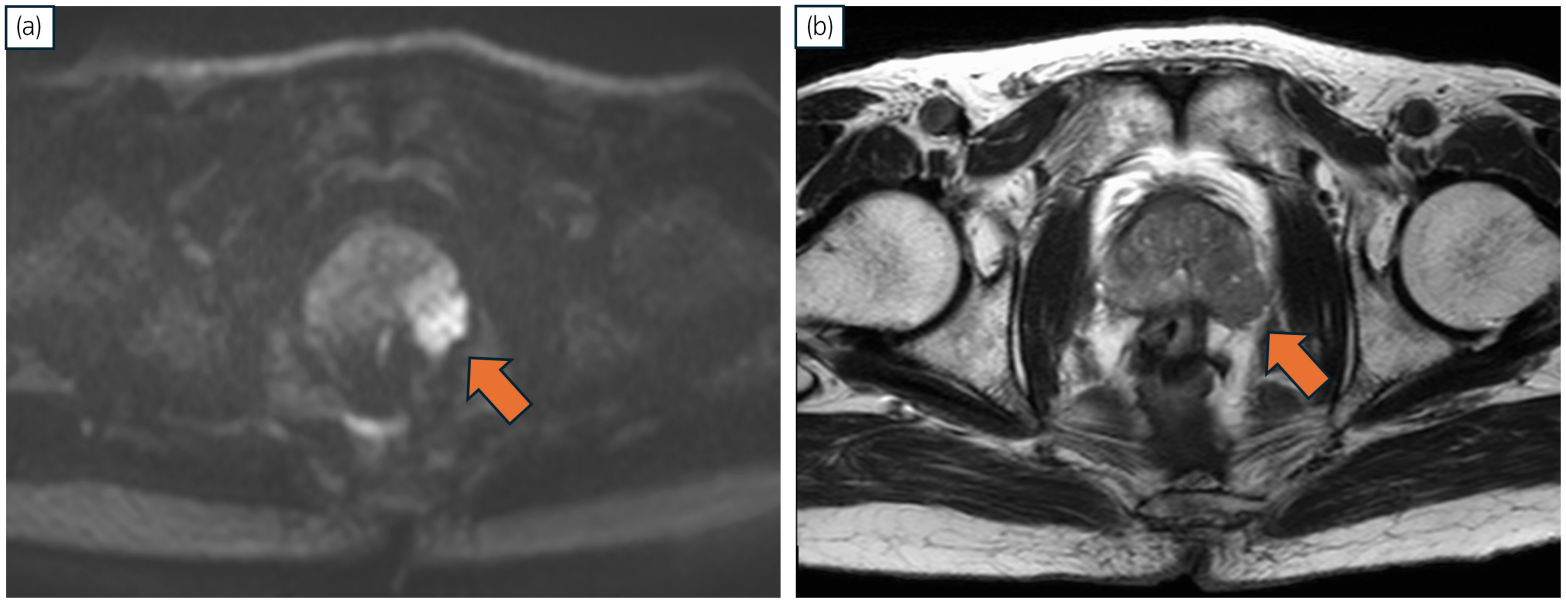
(a) Diffusion‐weighted and (b) T2‐weighted magnetic resonance images revealed extracapsular extension at the time of initial diagnosis.
